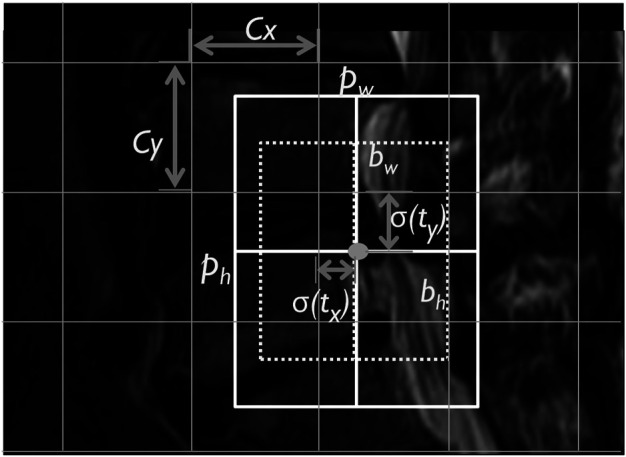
The lumbar MRI bounding box YOLOv3 predicted box image. *Note*. C_x_, C_y_, corner: p_w_, p_h_, bounding box width and height; b_w_, b_h_, predicted width and height; and $\sigma \left(t_{x}\right)$, $\sigma \left(t_{y}\right)$, sigmoid function.
